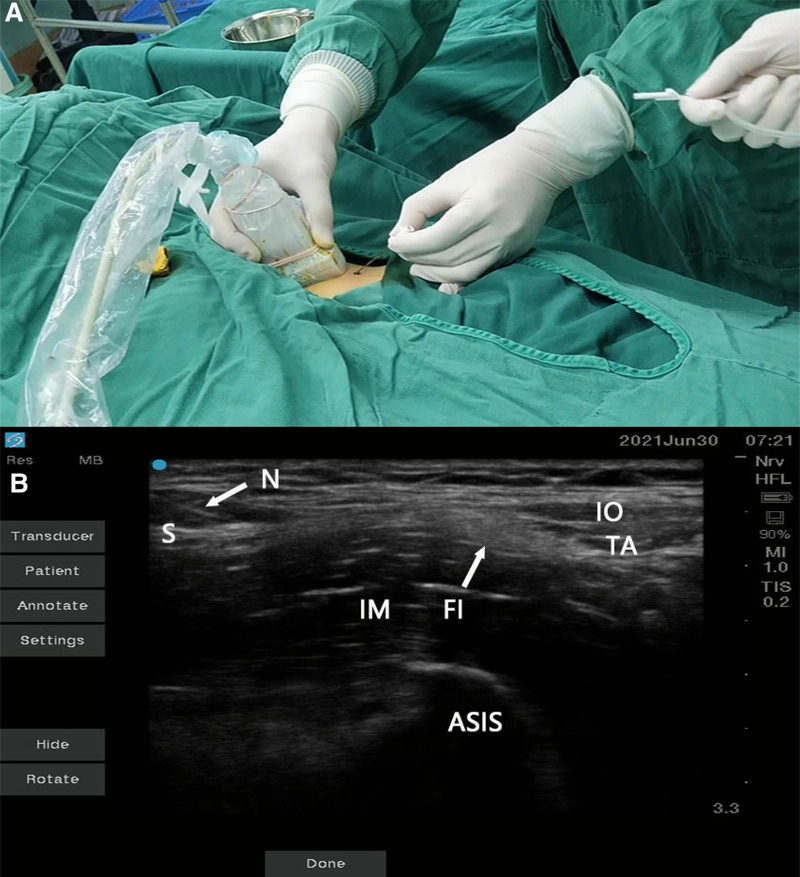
Ultrasound probe placement and imaging for fascia iliaca compartment block. (A) Positioning of the high-frequency ultrasound probe perpendicular to the inguinal ligament over the middle and outer thirds, with the patient in the supine position. (B) Ultrasound image displaying the “hourglass sign,” delineating the IO, TA, the S, and the IM. IM = iliacus muscle, IO = internal oblique, TA = transversus abdominis, S = sartorius muscle.
